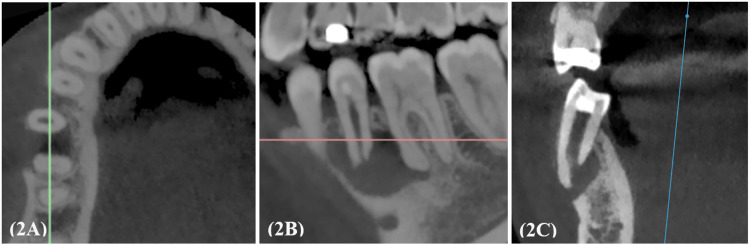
Cone-beam computed tomography (CBCT) 2A-2C: Periapical radiolucency associated with tooth 45 visualized in axial, sagittal, and coronal planes, respectively, demonstrating the extent of the lesion.
